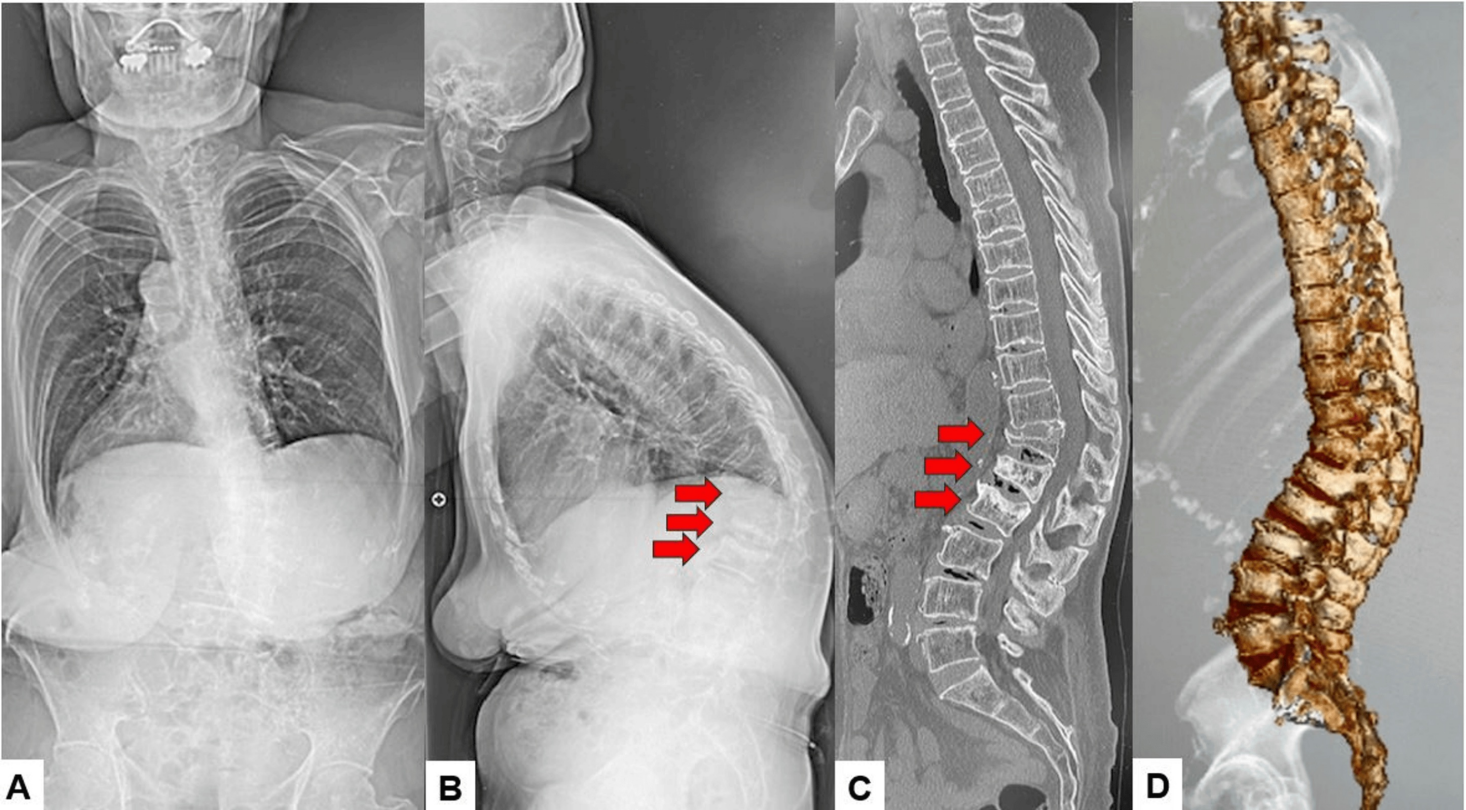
Preoperative radiograms A: Posteroanterior whole spine radiogram, B: Lateral whole spine radiogram, C: Mid sagittal reconstruction CT, and D: 3D CT. Red arrows indicate osteoporotic vertebral fractures.
